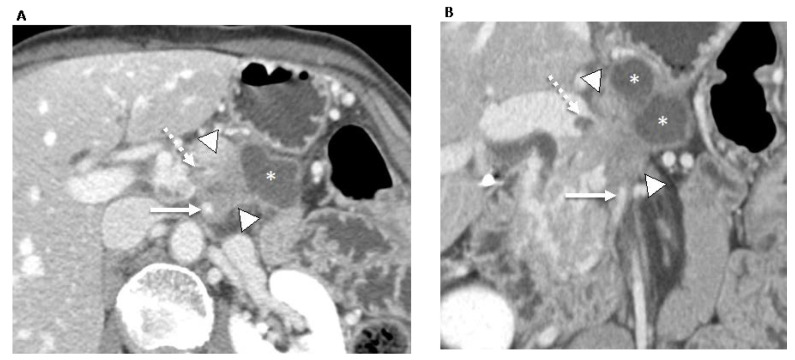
70-year-old man with locally advanced pancreatic ductal adenocarcinoma. Contrast-enhanced CT in the axial (A) and coronal (B) planes in the portal venous phase shows an ill-defined pancreatic neck and body tumor (arrowheads) with an adjacent pseudocyst (asterisks). The tumor involves the locoregional vessels including encasement (>180°) of the superior mesenteric artery (white arrow) and superior mesenteric vein/portomesenteric junction, which was severely narrowed with small non-occlusive thrombus (dashed arrow). The patient underwent palliative radiotherapy.
